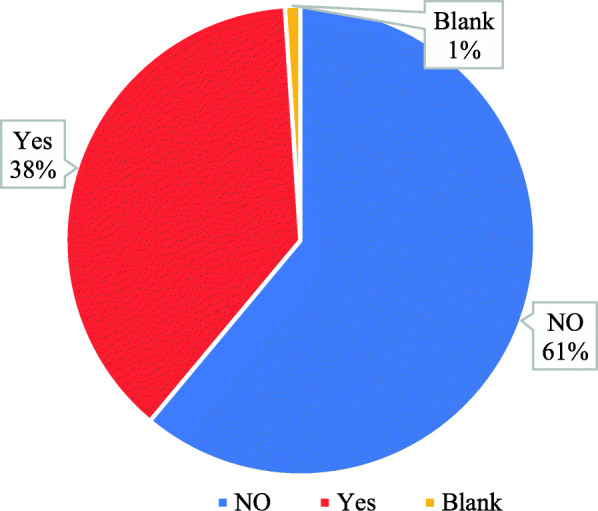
Subspeciality CT scan courses taken by the participants
